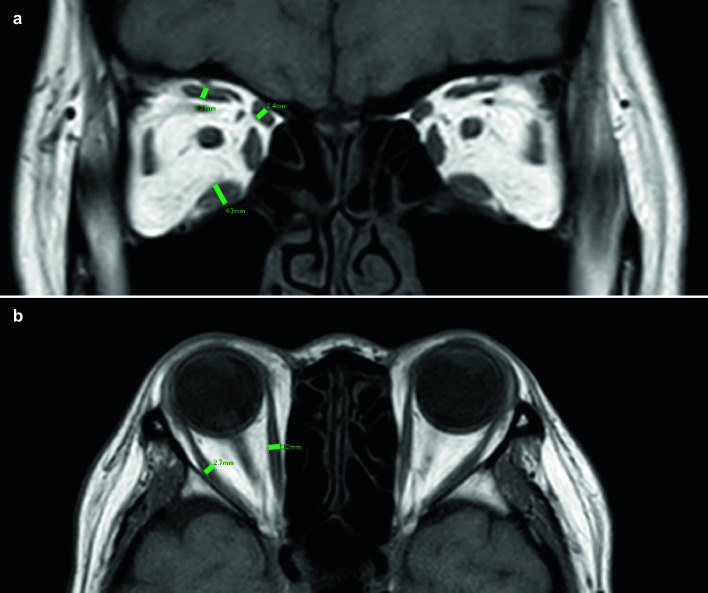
Measurement of extraocular muscle thickness. a The thicknesses of the SR, IR, and SO were measured on coronal sections of orbital MRI as the short axis of the thickest part in each muscle. b The thicknesses of the MR and the LR thickness were measured on horizontal sections of orbital MRI. *SR* superior rectus muscle, *IR* inferior rectus muscle, *LR* lateral rectus muscle, *MR* medial rectus muscle, *SO* superior oblique muscle, *MRI* magnetic resonance imaging
